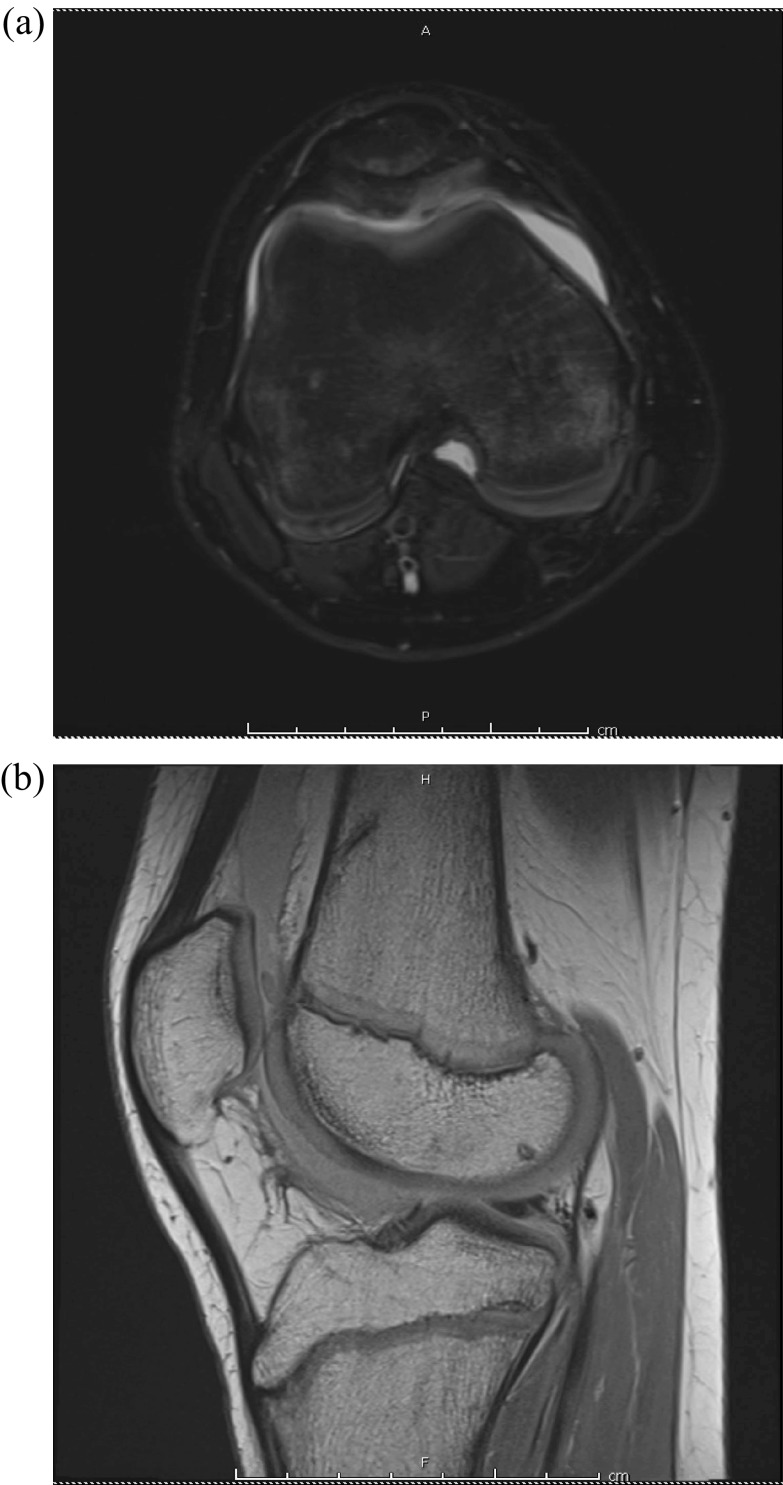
Axial T2- and sagittal proton density-weighted MRI showing incorporation of the previously fixed chondral fragment with near anatomic articular surface (a and b).
